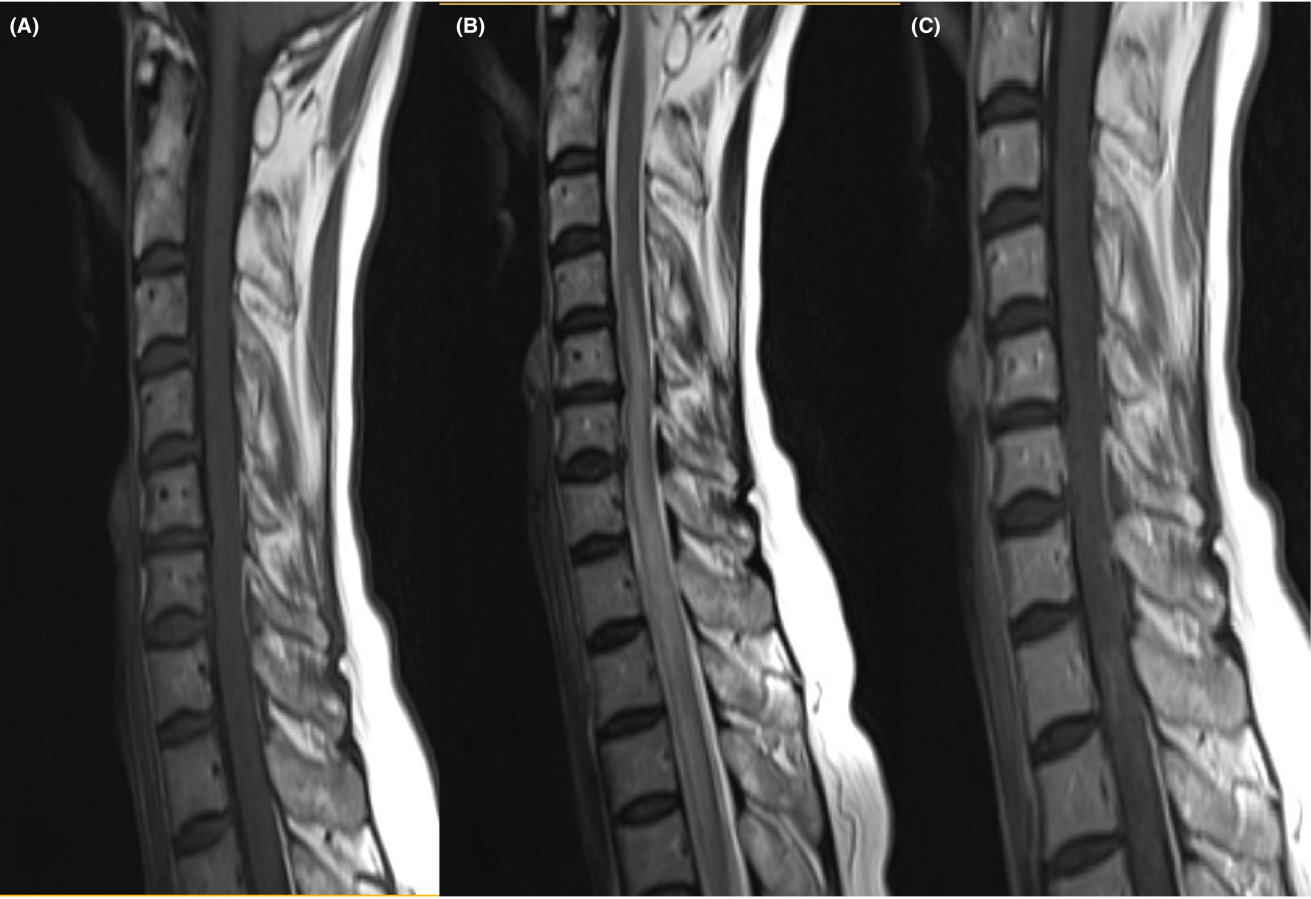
Cervicothoracic MRI scans show a long segment T1 iso signal (A) and T2 high signal (B) intensity between C4 and T5 level in the cervical cord with extension to the thoracic cord, with faint enhancement in some parts in the post contrast images (C).
